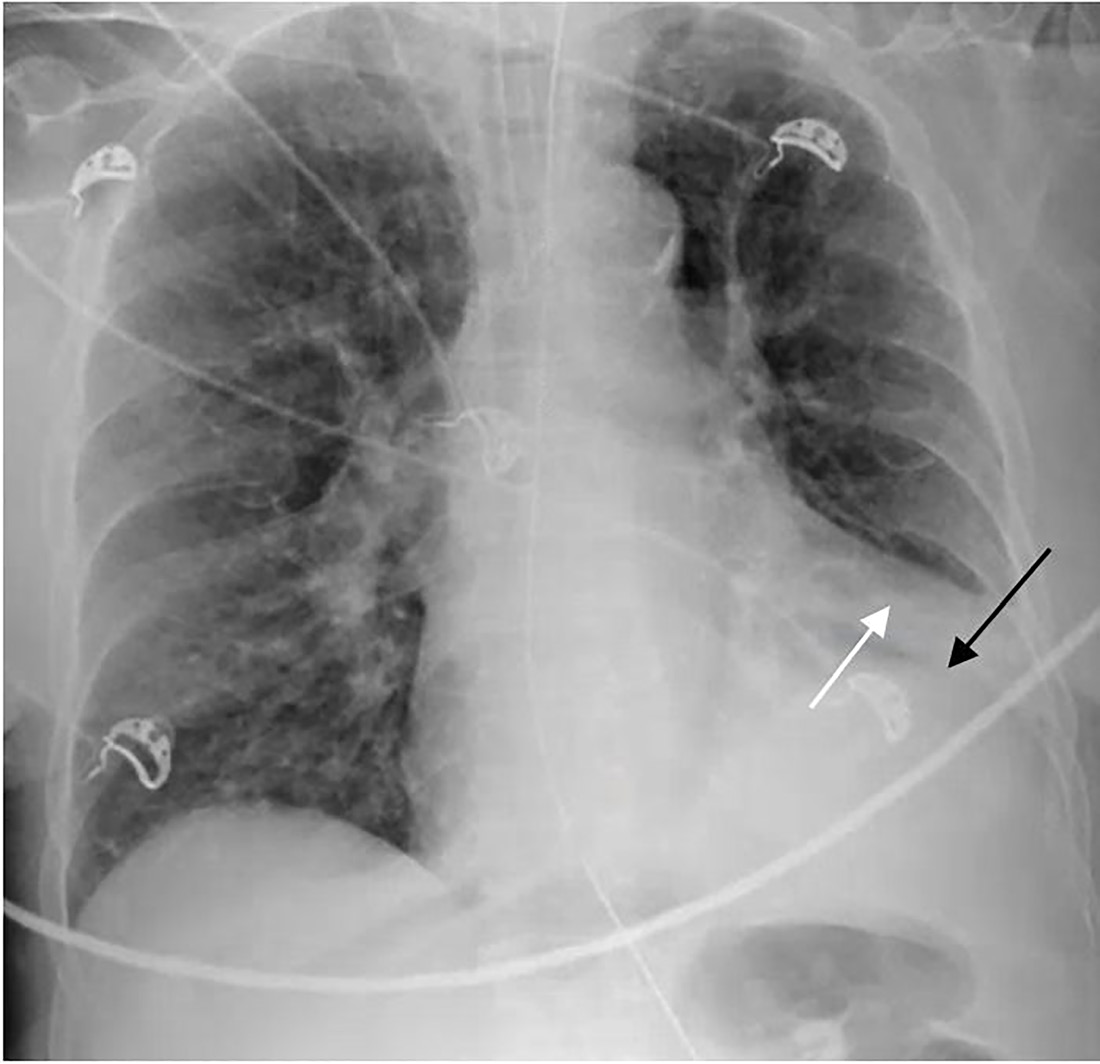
Posterior-anterior radiograph of the chest demonstrating elevation of left hemi-diaphragm (black arrow) and left lower lobe consolidation (white arrow).
